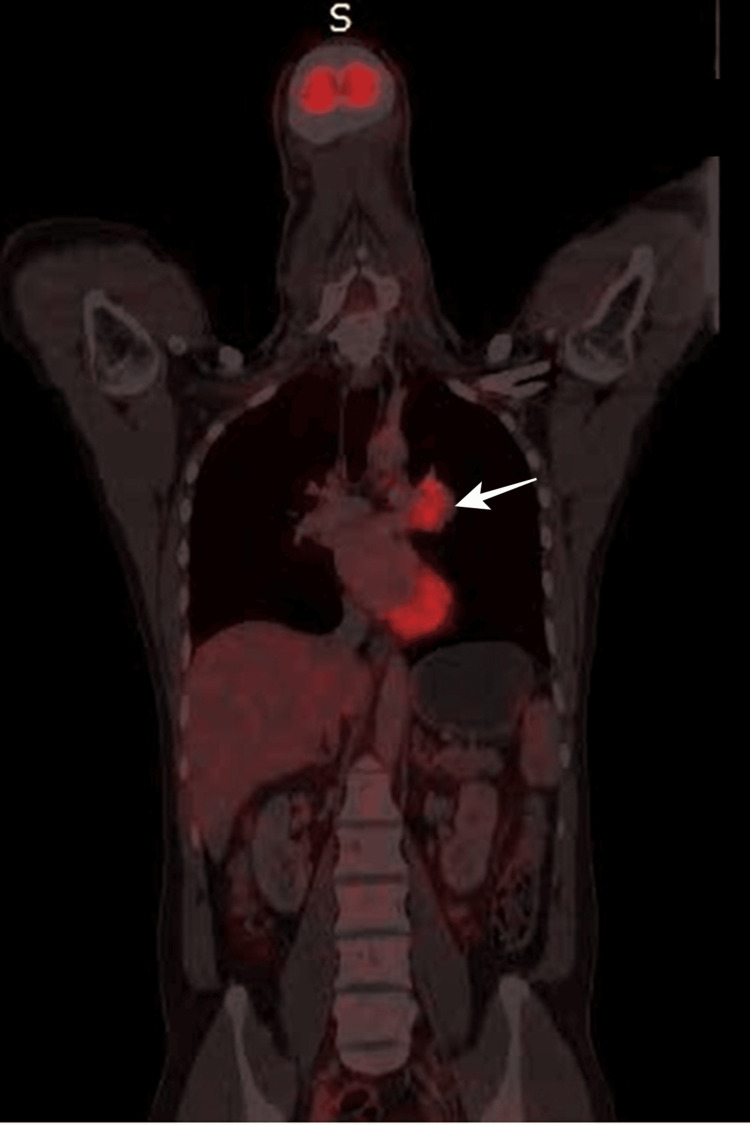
Whole-body PET-CT shows an Isolated FDG avid mass (white arrow) in the left hilum of lung FDG: fluorodeoxyglucose
